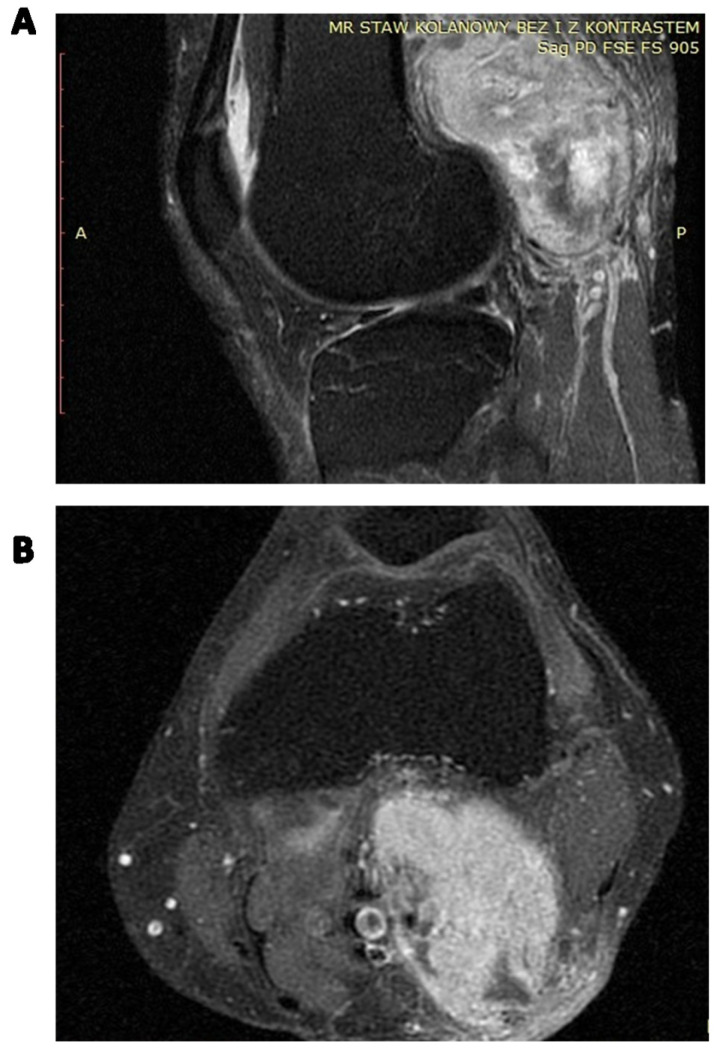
Contrast-enhanced MRI of the locally advanced left popliteal fossa SFT abutting popliteal vessels: (A) coronal image; (B) axial image.
